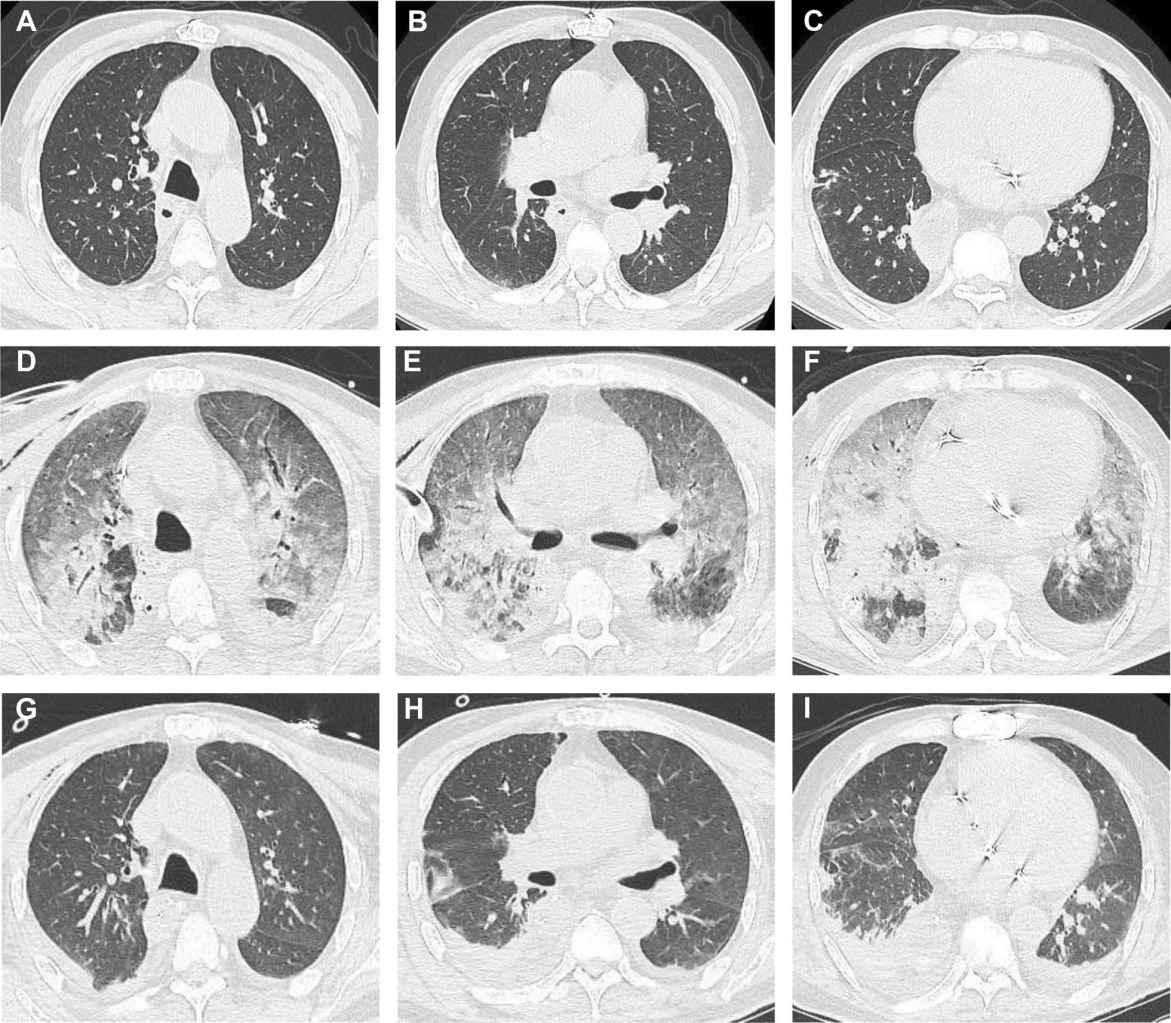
The changes of chest CT. A–C on hospital admission. D–F 8 days after operation when the patient was reintubated. G–I 14 days after operation and 6 days after reintubation
